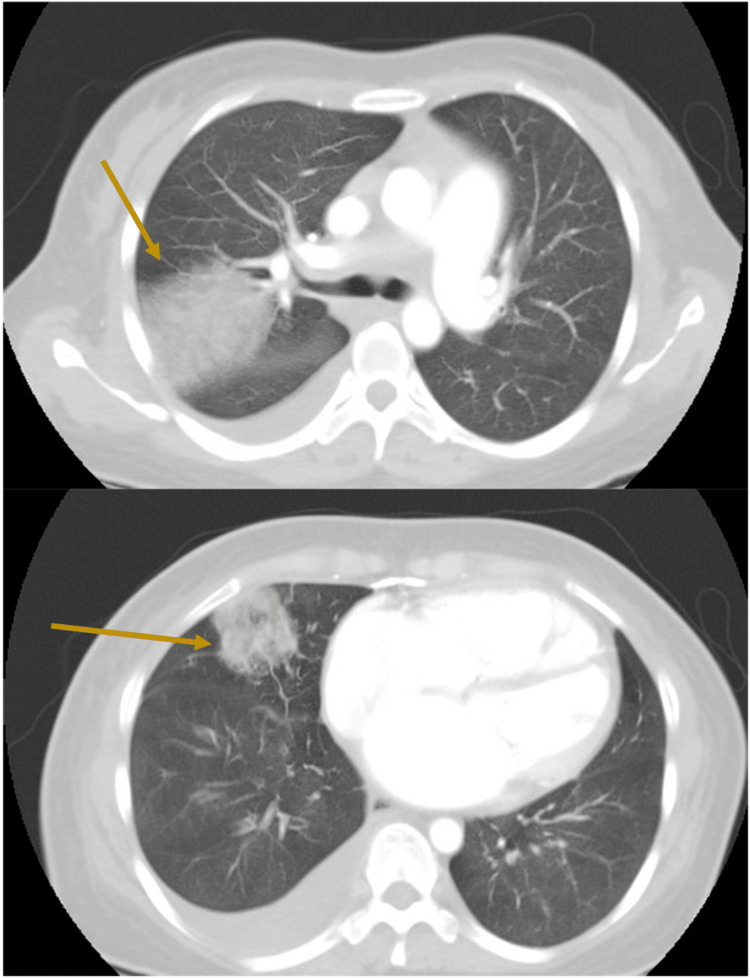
CT chest angiography Showing alveolar haemorrhage and enlarged cardiac size.
